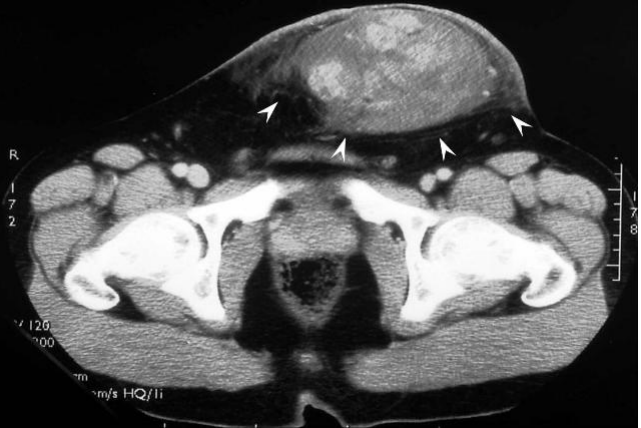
Computed tomography depicted a solid tumor with heterogeneous contrast enhancement (arrowheads) in the adipose tissue.
